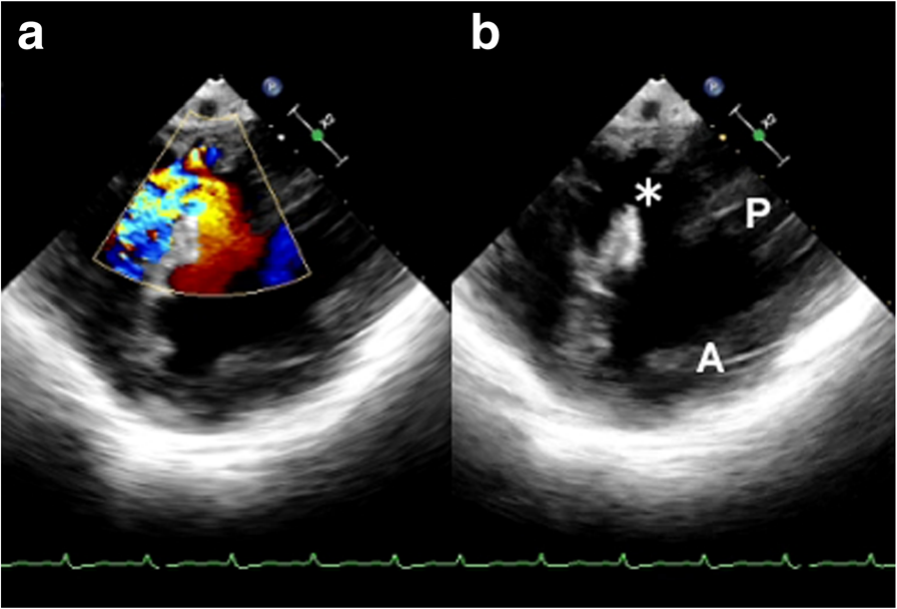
The preoperative transesophageal echocardiogram. a It showed transseptal interventricular communication with a shunting ratio of 2.4. b The defect (asterisk) located in the posterior septum close to the base of the posteromedial papillary muscle (P). A: anterior papillary muscle
